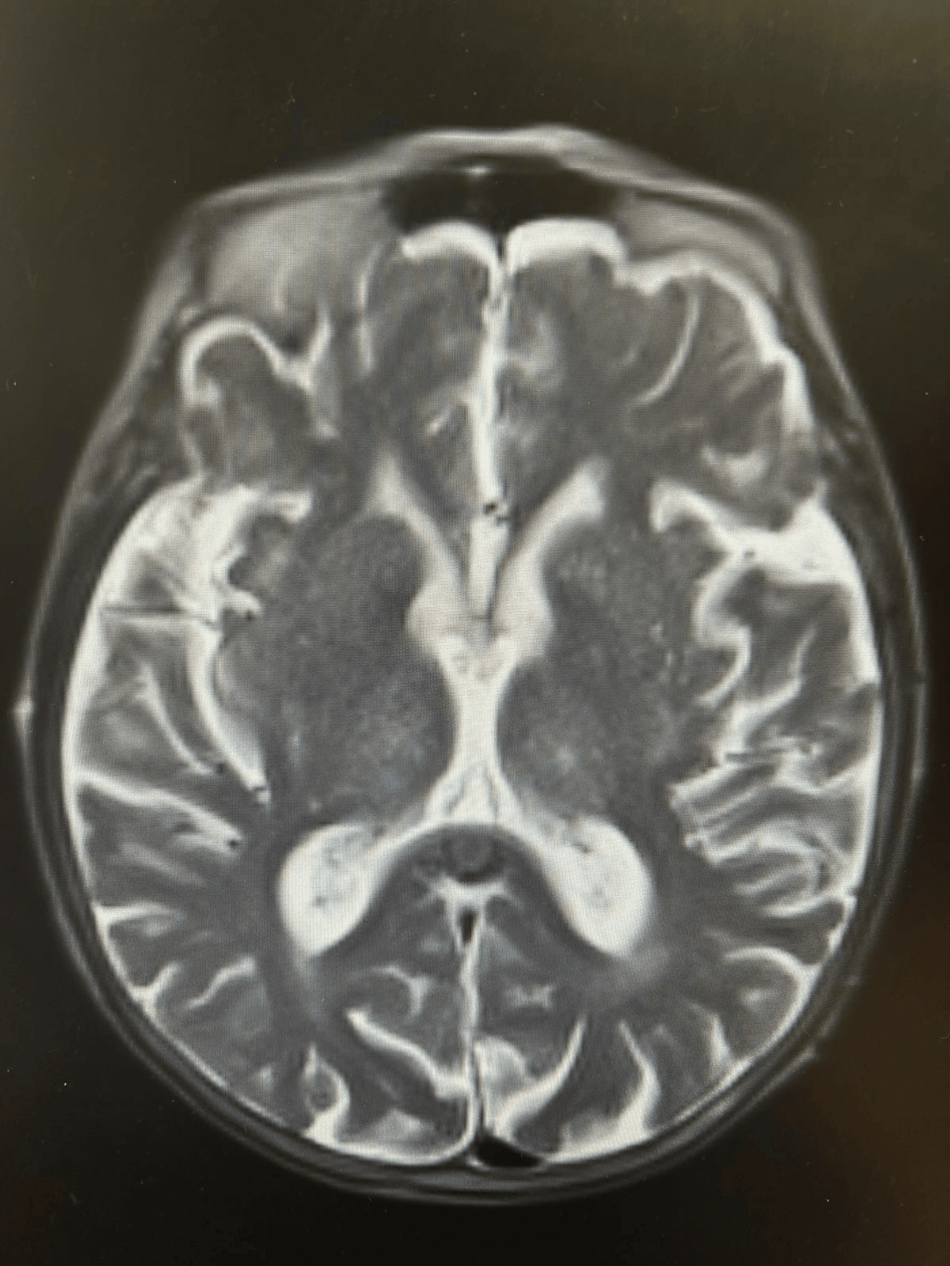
Axial view of the brain MRI scan An unremarkable brain MRI scan with evidence of age-related brain involutional changes. MRI: magnetic resonance imaging
